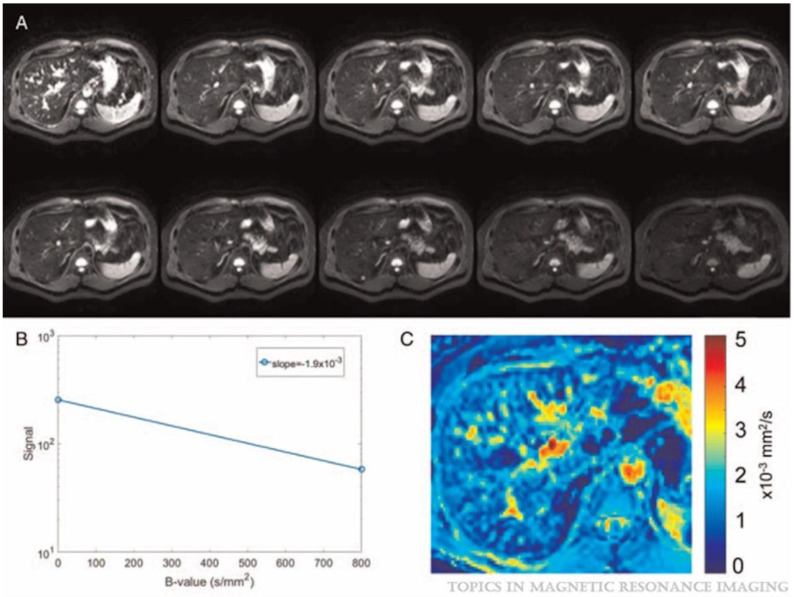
DWI acquired (A) with 10 B values (b = 0, 10, 20, 30, 40, 50, 100, 200, 400, and 800 s/mm^2^). (B) The signal from the two extreme b-values is plotted semi-logarithmically as a function of their b value and a linear regression is used to extract the ADC (mm^2^/s) for a specific pixel. (C) A color parametric map of ADC values is generated for each pixel in the image [8].
